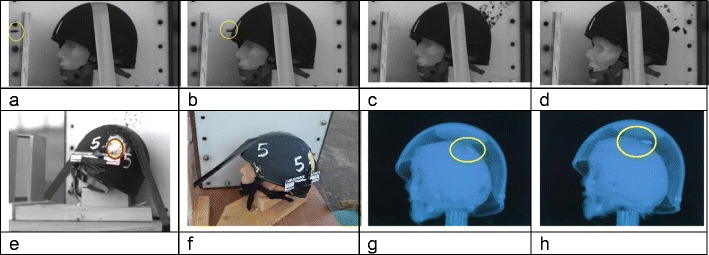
a–d Impact sequence model 1, frames from V12 camera. a Bullet (circled) prior to cutting foil. b Helmet impact, bullet circled. c Shower of paint from bullet impact inside rear of helmet. d Distortion of face due to stretching from temporary cavity development. e Impact of bullet inside rear of helmet 5. Distortion of helmet material from bullet impacting sideways circled. Frame from V1212 camera. f Model 5 in situ after shooting. g–h Flash X-ray images. g Model 3. h Model 2. Bullets circled. Forensic scale visible in panels e and f.
